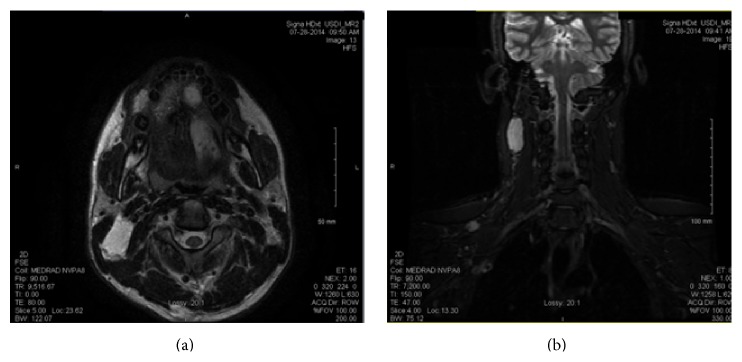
(a) Axial T2-weighted MRI demonstrating soft tissue masses with hyperintense signal of the left tongue and right submandibular triangle. (b) Coronal T2-weighted MRI demonstrating hyperintense lesions of the right suprahyoid posterior triangle and right supraclavicular fossa.
